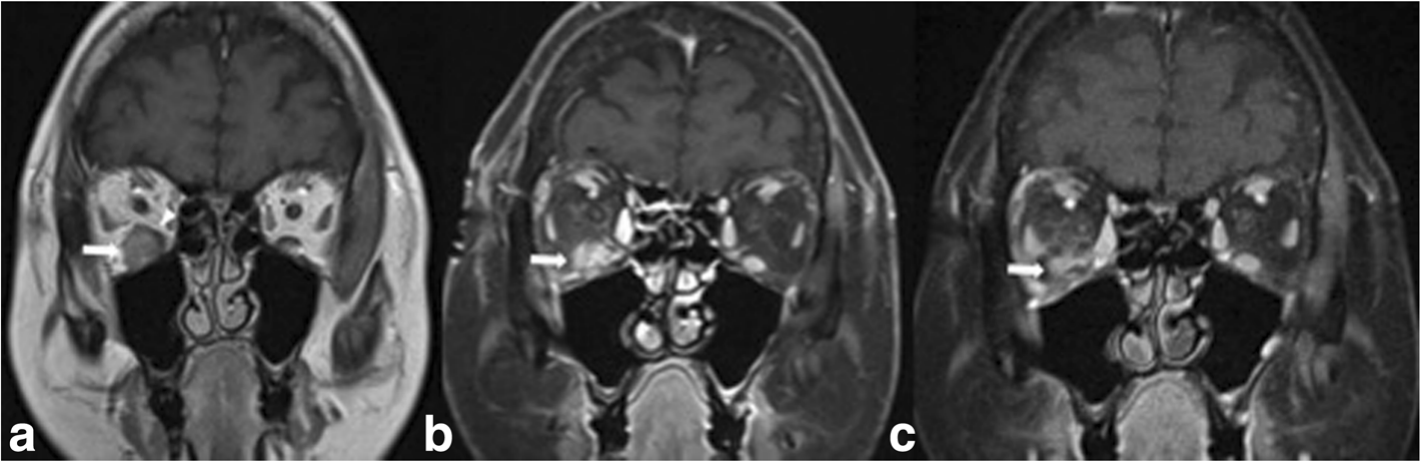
a T1-weighted MR image depicting the lesion at the time of first diagnosis, as a slightly hyperintense solid nodule (arrow) located on the floor of the right orbit. Part of the belly of the inferior rectus muscle appears compressed and displaced (arrowhead). Post-contrast images were not acquired in this MR examination. b Post-contrast fat-suppressed T1-weighted image shows the shrinkage of the lesion (arrow) 2 months after the initiation of orbital radiotherapy followed by fulvestrant-based hormonotherapy. c Post-contrast fat-suppressed T1-weighted image shows further shrinkage of the lesion (arrow) 17 months after the initiation of radiotherapy + hormone therapy. Palbociclib was also added between B and C, see text for details). Right inferior rectus muscle is not discernible in (b) and (c). The maximum dimension of the lesion was 14 mm in A, and decreased to 12 mm In B and to 7 mm in (c)
